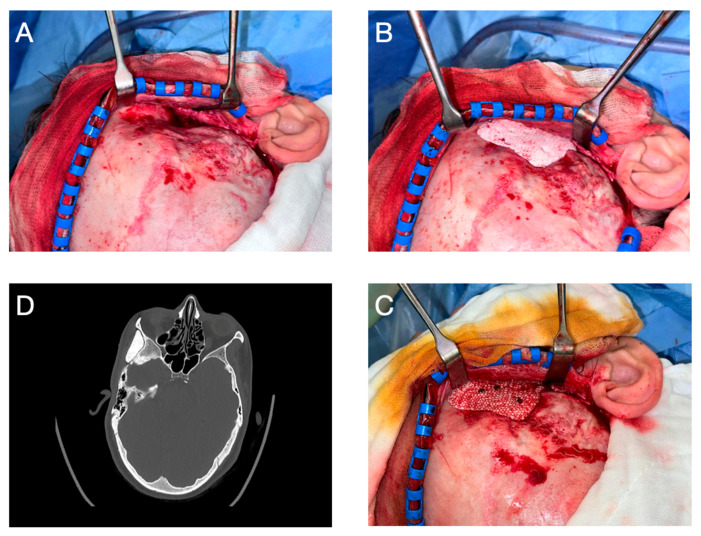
Key surgical steps and postoperative surgical assessment: Exposure of the entire right temporal fossa via a hemicoronal approach (A), trial fitting of the PSI (B), and fixation of the PSI using three 2.0 osteosynthesis screws (C). Correctly positioned PSI in the postoperative CT scan (D).
